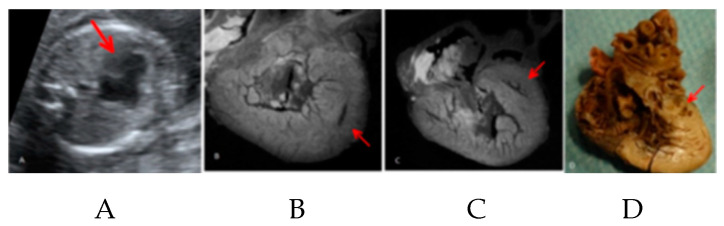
The diagnostic ability of micro-CT with prenatal fetal echocardiography and autopsy in a case of hypoplastic left heart syndrome. (A) Prenatal ultrasound: four chambers view shows the hypoplastic mitral valve and left heart (red arrow); (B) postmortem micro-CT at the level of the ventricle compares the right ventricle with the hypoplastic left ventricle (red arrow); (C) four-chamber view at postmortem micro-CT shows the hypoplastic left ventricle (red arrow); (D) four-chamber view at conventional autopsy shows the hypoplastic left ventricle (red arrow) [29].
